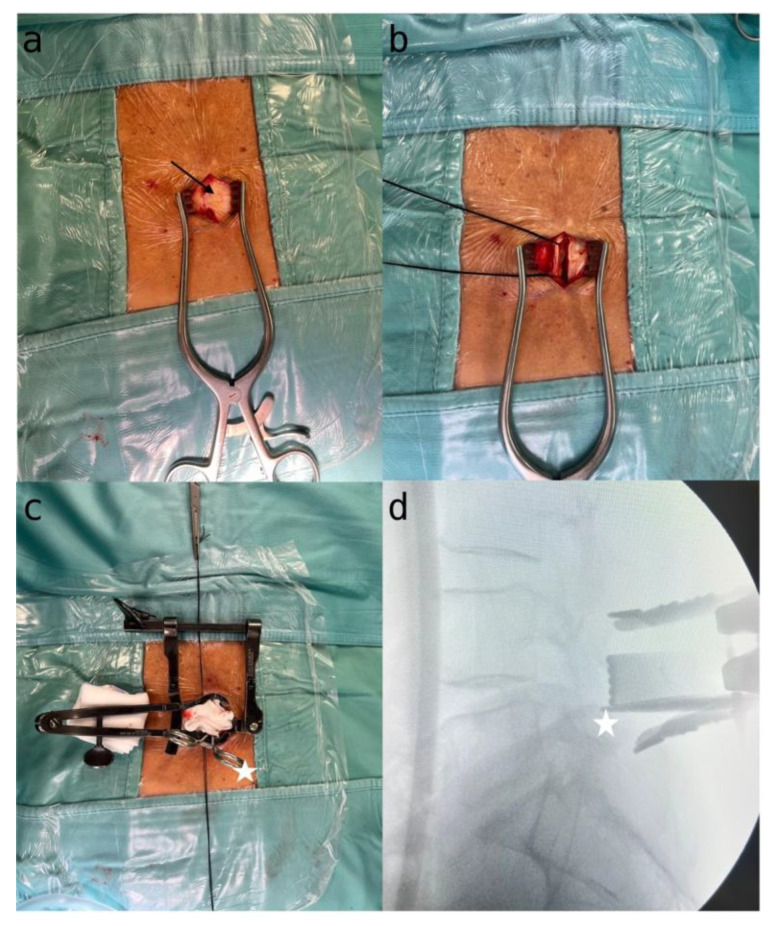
(a) Exposure of the skin incision and the muscular fascia (black arrow); (b) handling of the muscle fascia; (c) skeletonization of the homolateral lamina and positioning of a Caspar distractor. Klemmer forceps (white star) placed beneath the lamina for X-ray verification; (d) X-ray confirmation of the Caspar distractor’s accurate placement at the correct level.
